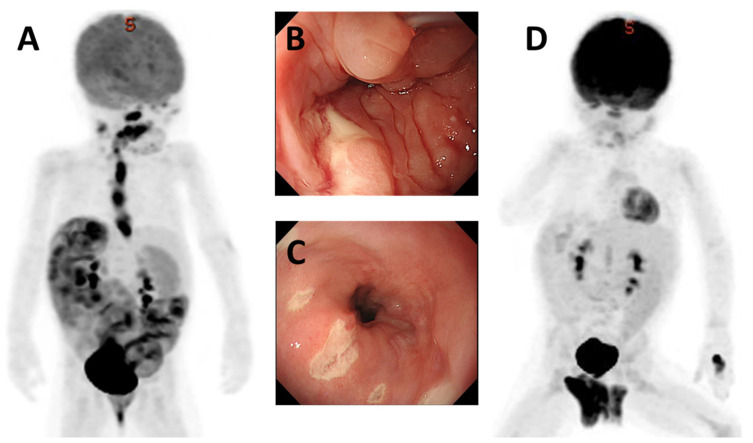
EBV-positive mucocutaneous ulcer (EBVMCU). Case 1. A girl of 1 year and 5 months of age. At 8 months after LDLT, she developed gastrointestinal symptoms, including abdominal pain and watery stool, with fever. (A) PET-CT showed numerous hot spots, especially in the esophagus. (B,C) Upper endoscopy revealed numerous ulcerative lesions in the esophagus, and biopsy revealed EBVMCU. (D) Her symptoms resolved within 2 weeks after dose reduction of her immunosuppressive drugs, and PET-CT showed the disappearance of the hot spots of the gastrointestinal tract.
